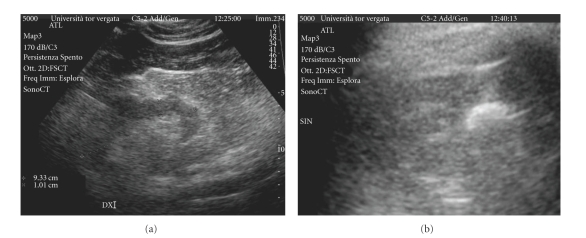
Ultrasonography imaging: normal right kidney (a) and absence of left kidney in the renal space (b).
